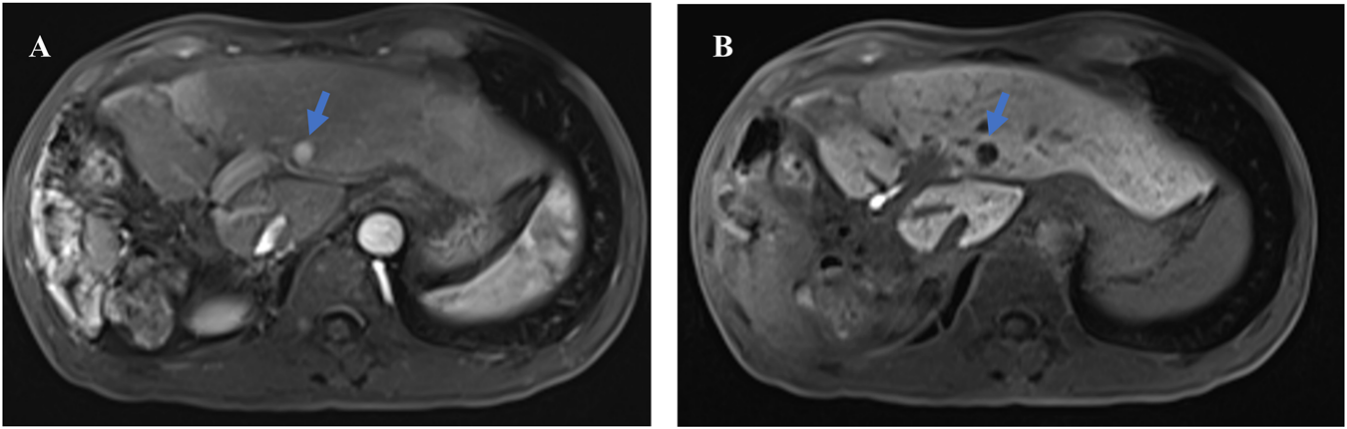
Follow up with tumor recurrence. A A 55-year-old male patient, axial follow-up MRI taken 3 months after ALPPS Stage 2 showed an obvious hyperenhancement nodule (arrow) in the arterial phase: suggestive of recurrence. B The same 55-year-old male patient, axial follow-up MRI taken 3 months after ALPPS Stage 2 showed an obvious hypointensity nodule (arrow) in the hepatobiliary phase, indicating intrahepatic recurrence that requires early local therapy
